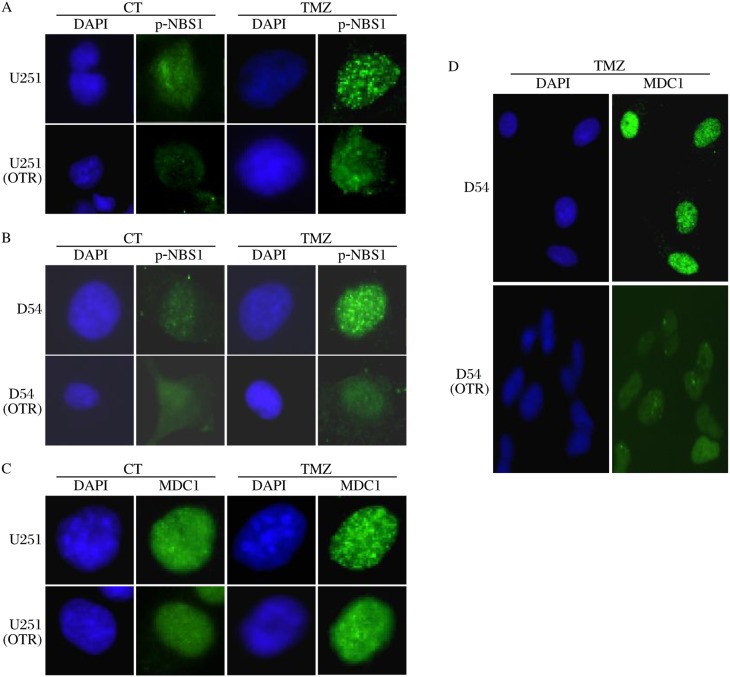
The resistant GBM cells show defective DDR. Phosphorylated NBS1 (p-NBS1) (A and B) and MDC1 (C and D) form distinct foci 72 h post treatment in D54 and U251, but not in their TMZ-resistant counterparts. CT: control, TMZ: temolomide.
